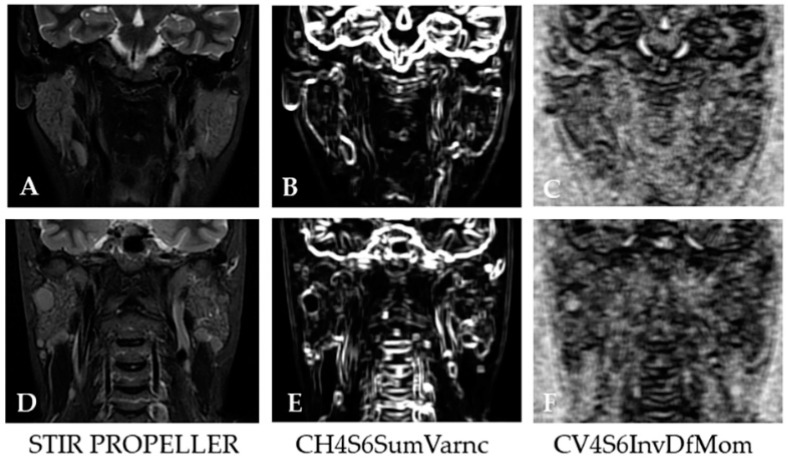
Texture maps presenting the distribution of the independent features (CH4S6SumVarnc, CV4S6InvDfMom) associated with NHL development in PG of patients with pSS on the selected MRI coronal STIR PROPELLER image. (A–C) images of a patient with pSS and without lymphomatous proliferation in the parotid gland; (D–F) images of a patient with pSS and NHL development in the right parotid gland.
